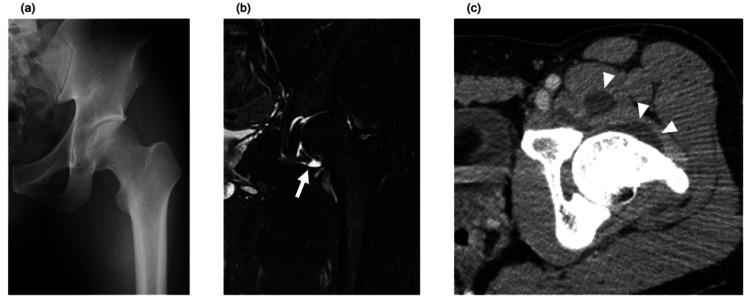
Imaging findings of the left hip during the clinical course (a) Anteroposterior plain radiograph obtained at initial presentation showing preserved joint space without obvious osseous abnormality. (b) Coronal STIR magnetic resonance image obtained at initial presentation demonstrating a left hip joint effusion (arrow). (c) Contrast-enhanced computed tomography obtained after symptom recurrence showing persistent joint effusion, capsular distension, increased synovial enhancement, and fluid extension toward the iliopsoas bursa (arrowheads). STIR: Short tau inversion recovery.
